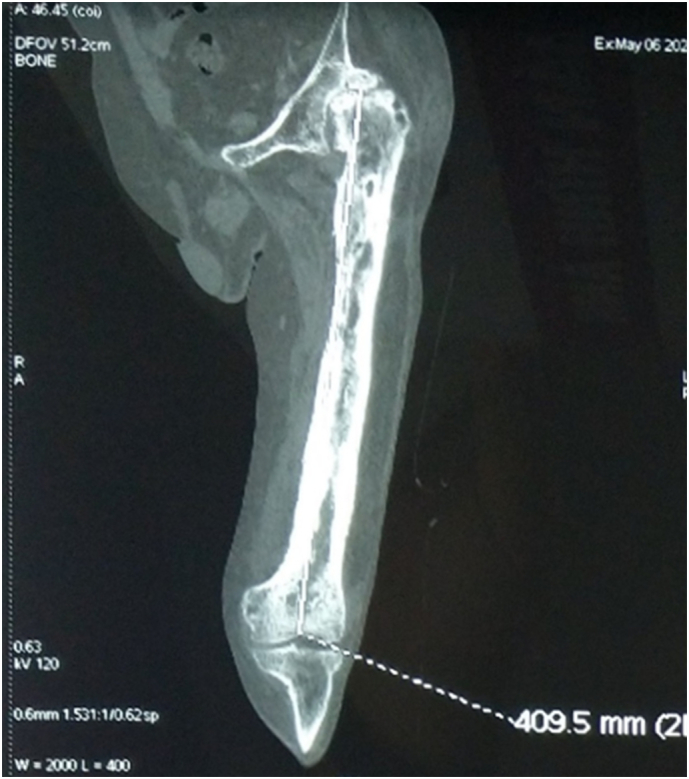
Imaging X-ray and CT-Scanner prior to operation.
